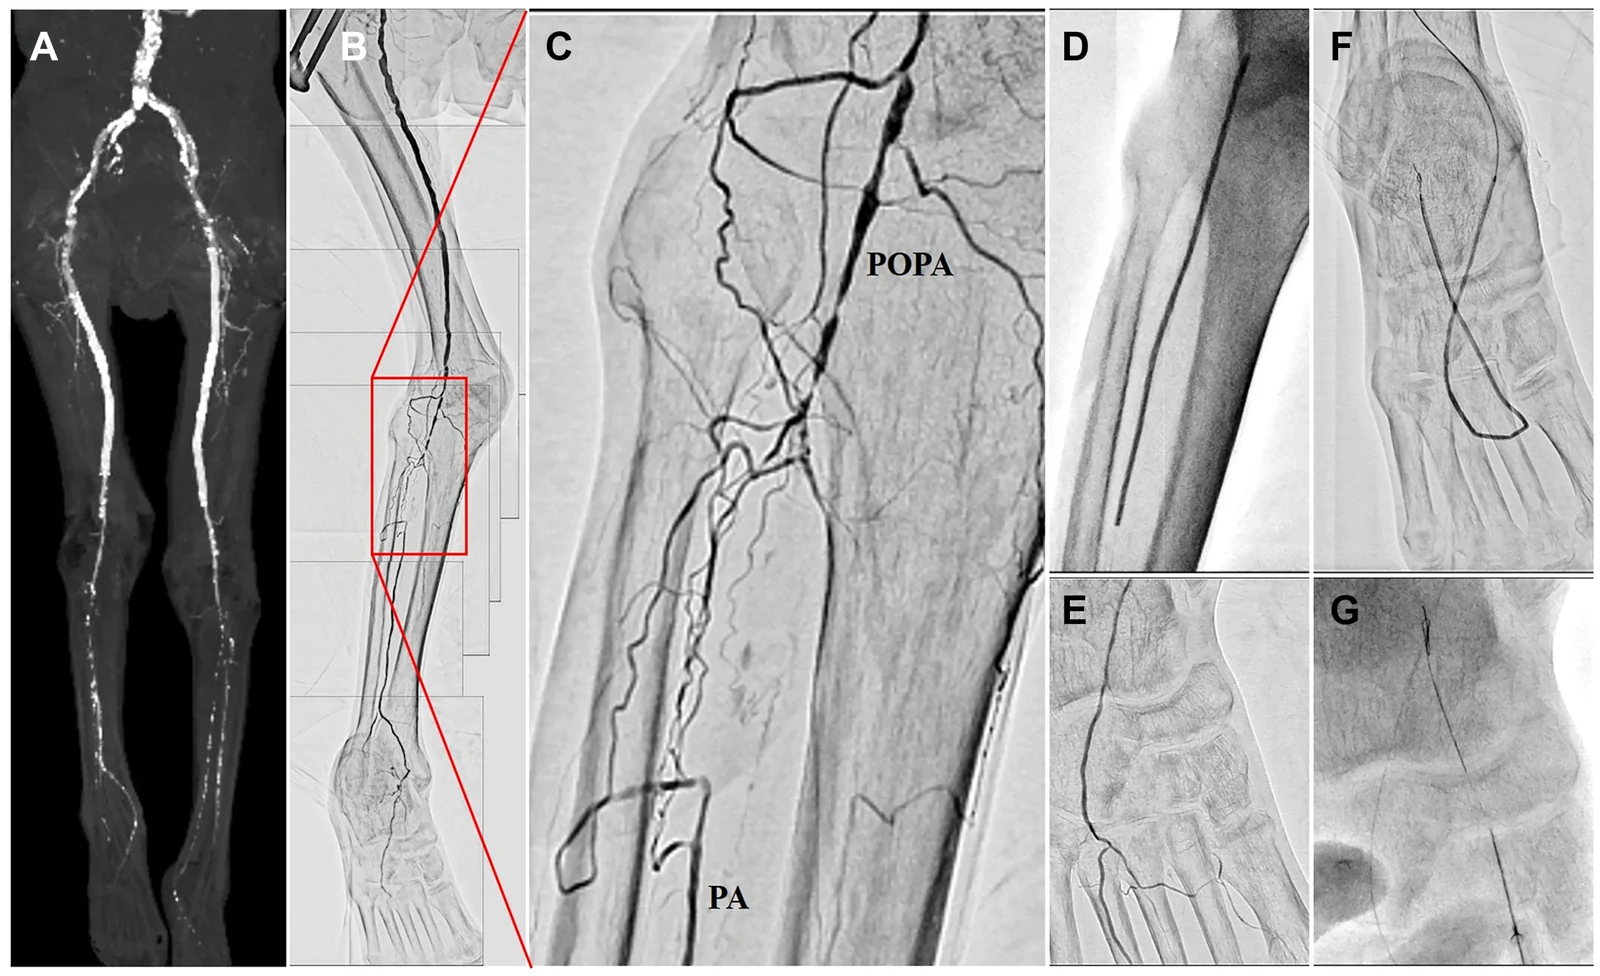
A, Computed tomography angiography depicting occlusions of the right anterior tibial artery (ATA) from its ostium (*arrow*) and the tibioperoneal trunk (TPT) with the type IIIA variant anatomy. B and C, Initial angiography showing diffuse stenoses of the popliteal artery (*POPA*) and occlusions of both the ATA and TPT. D, Balloon angioplasty of the occluded TPT. E, Angiography of the pedal arch. F, Pedal arch angioplasty. G, Puncture of the dorsal artery after pedal arch angioplasty. *PA*, Peroneal artery.
